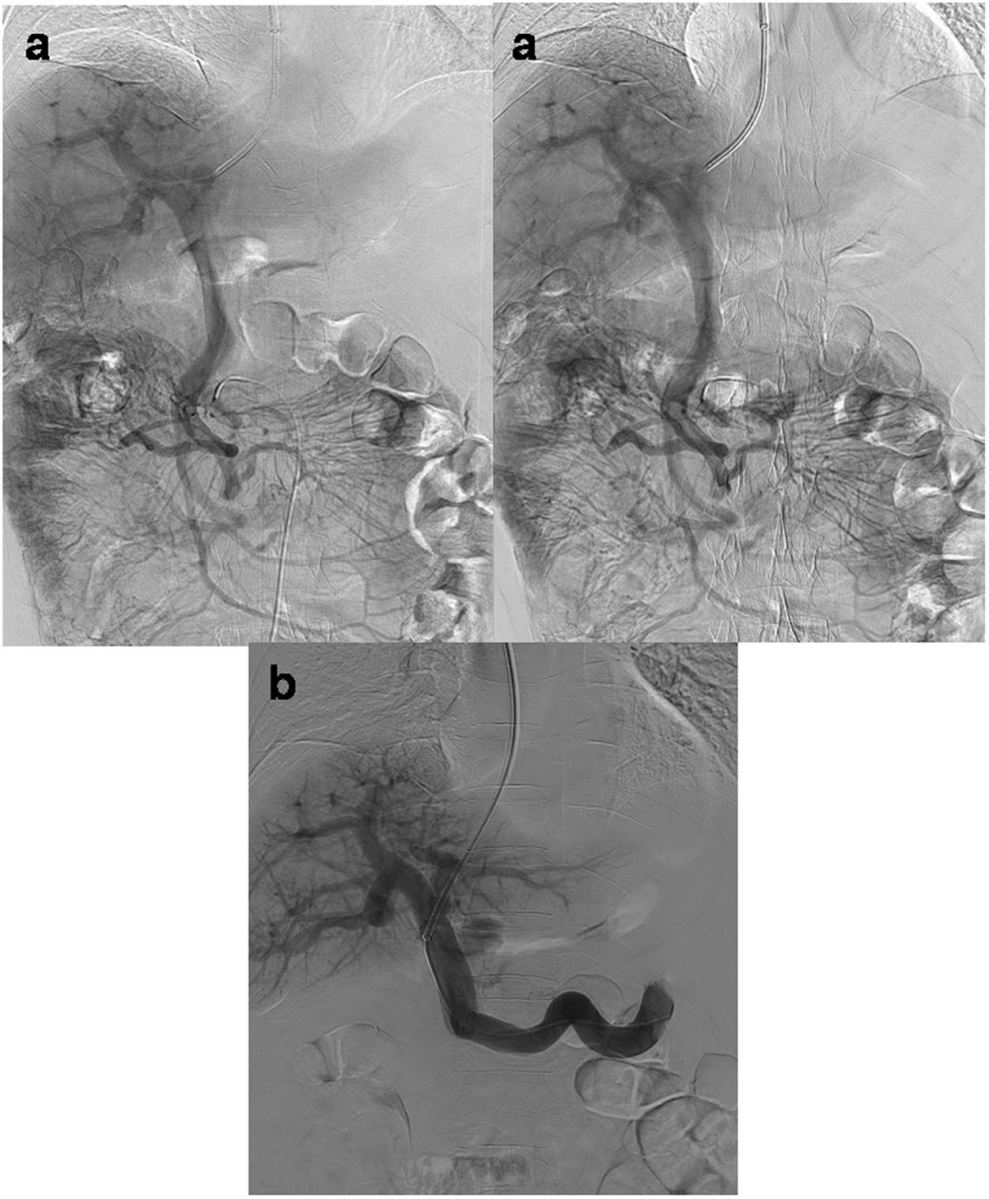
(a) In a 60-year-old man with TIPS placement, duplicate X-ray images of indirect portography at different rotation angles were horizontally combined to realize X-ray stereo vision to overcome repeated puncture failure. Observation with VR glasses produced stereo vision for easily identifying spatial relationships (the left portal vein was located at the left rear of the puncture needle). (b) After the puncture angle was adjusted toward the right rear, the puncture needle successfully entered the right portal vein. The subsequent portography confirmed that the needle had entered the right portal vein toward the right rear of the previous position.
